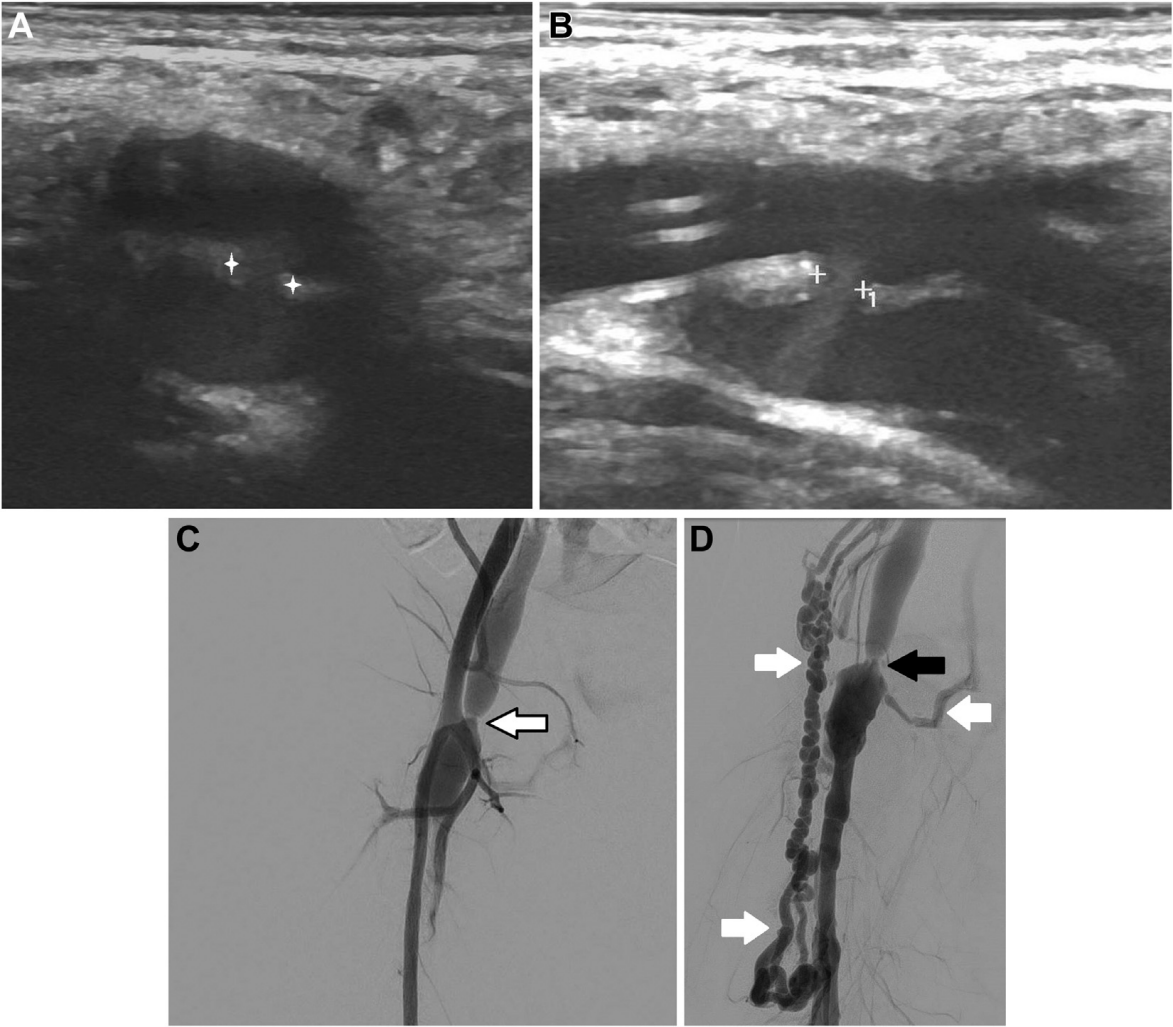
Imaging Findings (A) Transverse and (B) longitudinal grayscale ultrasound images demonstrating the side-to-side fistula between the dilated common femoral artery and posteriorly located vein measuring 1.6 mm. (C) Right iliac angiography: immediate shunting from the dilated right common femoral artery into an aneurysmal portion of the common femoral vein with proximal venous stenosis (arrow). (D) Ascending venography demonstrating severe stenosis of the right external iliac vein (black arrow) and collateral venous drainage in downward and upward directions (white arrows).
